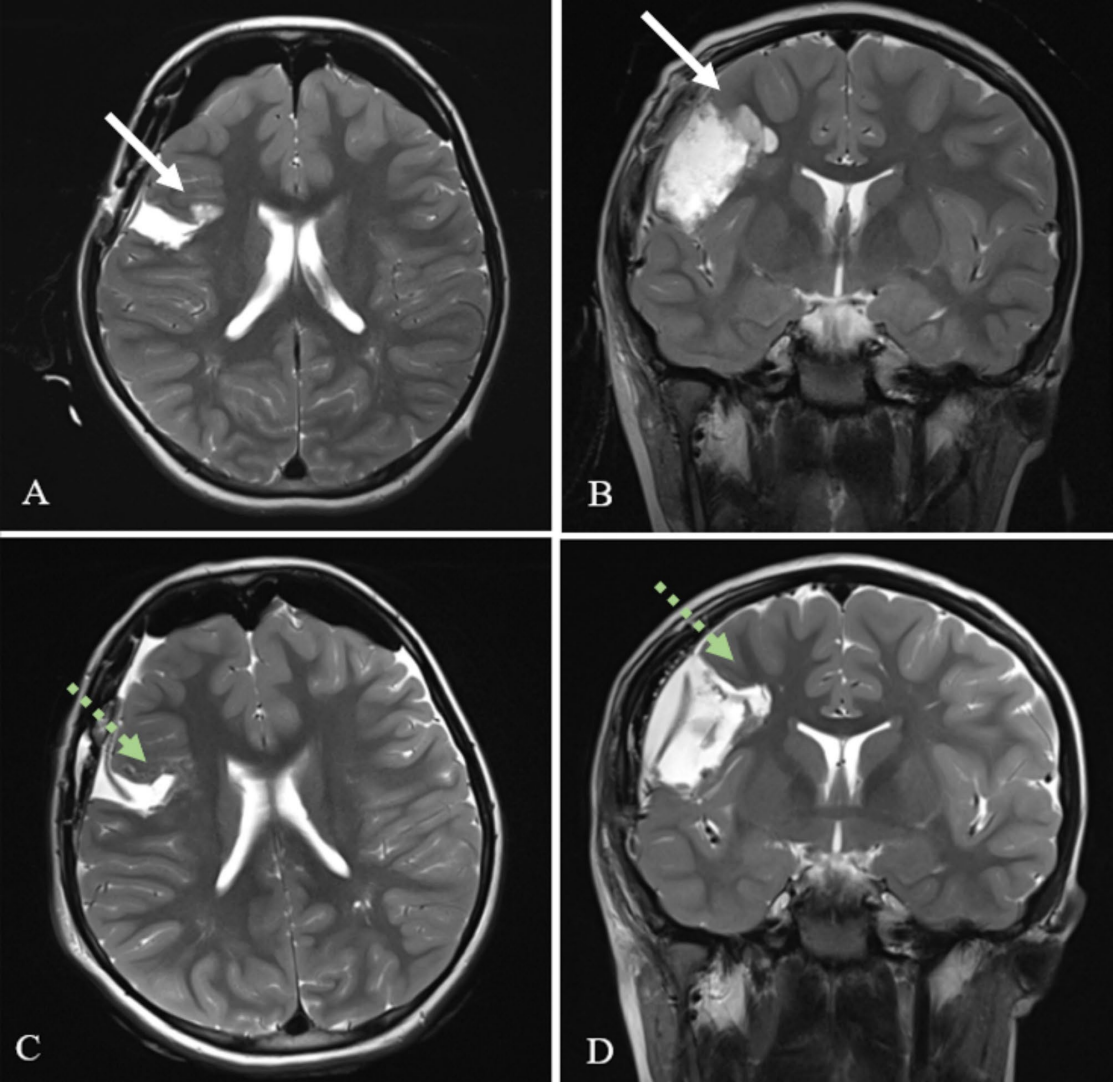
Intraoperative T2-weighted MRI images of a dysembryoplastic neuroepithelial tumor (DNET) in the right frontal lobe before (2 A-B) and after (2 C-D) continued resection following iMRI. Remaining tumorous tissue detected on iMRI before extended resection is demonstrated with white arrows (2 A-B). After resection, achieved GTR is demonstrated with green arrows (2 C-D)
